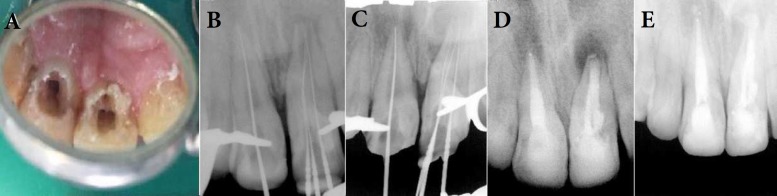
Prepared access cavities. Note that three orifices are detectable on tooth #9; *B, C)* Working length determination; *D)* Post treatment radiograph. Obturation was performed using MTA plug (6 mm of MTA) as apical barrier and backfilling with warm vertical technique; *E)* Follow up radiographs showed significant reduction in the size of apical radiolucencies on 3 months after treatment
